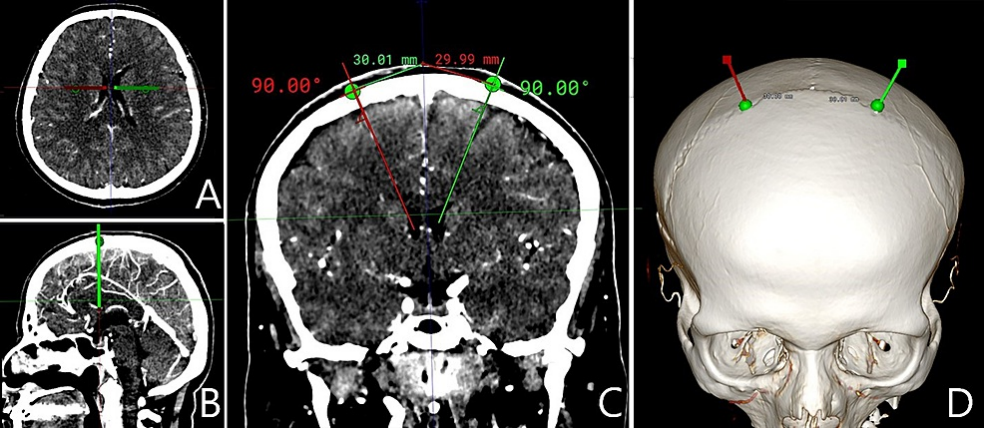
Entry point and EVD placement angle measurements on CT scan A, axial slices; B, trajectory of EVD insertion in the sagittal plane; C, trajectory of EVD insertion in the frontal plane; D, 3D reconstruction imaging depicting the point of trephination on the skull surface EVD = external ventricular drain; CT = computed tomography
